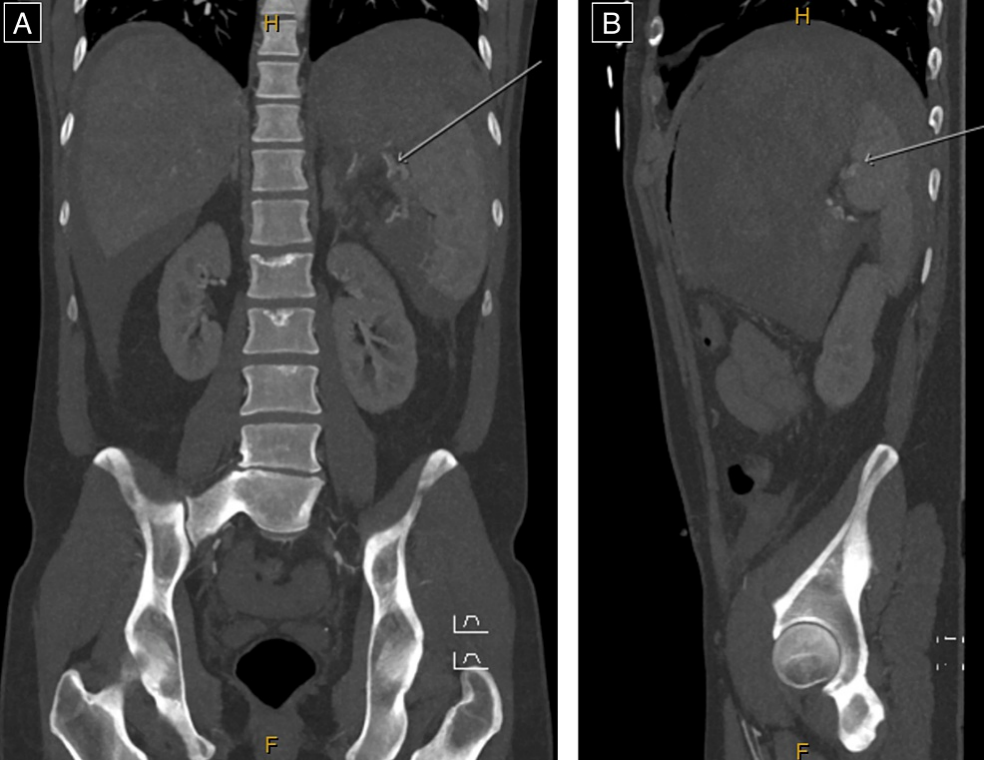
CT angiogram images demonstrating possible small splenic artery pseudoaneurysm (note arrows) at the splenic hilum on (A) coronal and (B) sagittal sections. CT: computer tomography.
